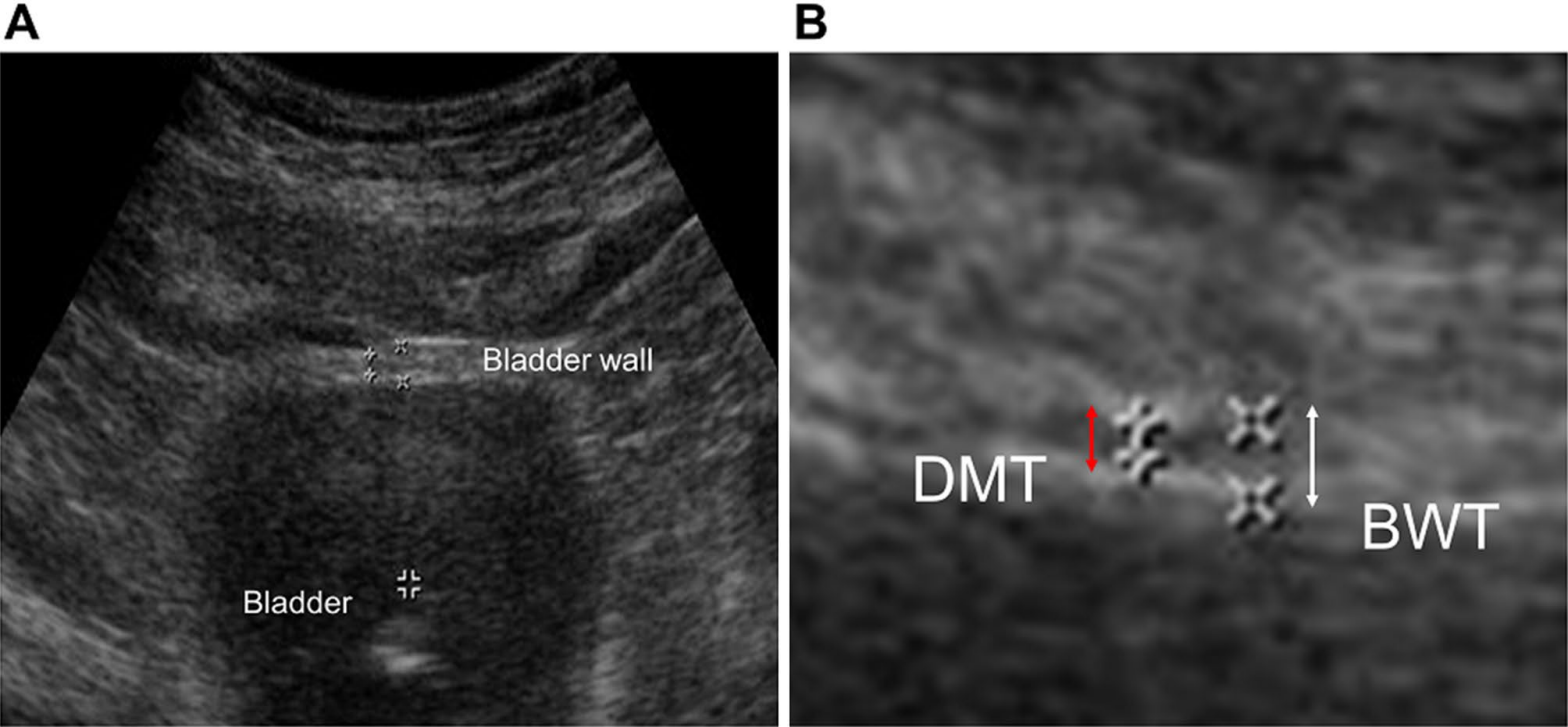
(A) Ultrasonography to measure the thickness of detrusor muscle and bladder wall, and (B) magnification to measure thickness. *DMT* detrusor muscle thickness, *BWT* bladder wall thickness.
